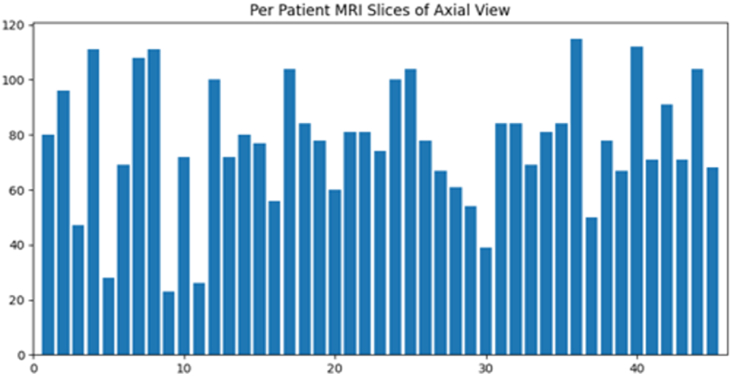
Axial view MRI slices of each patient.
